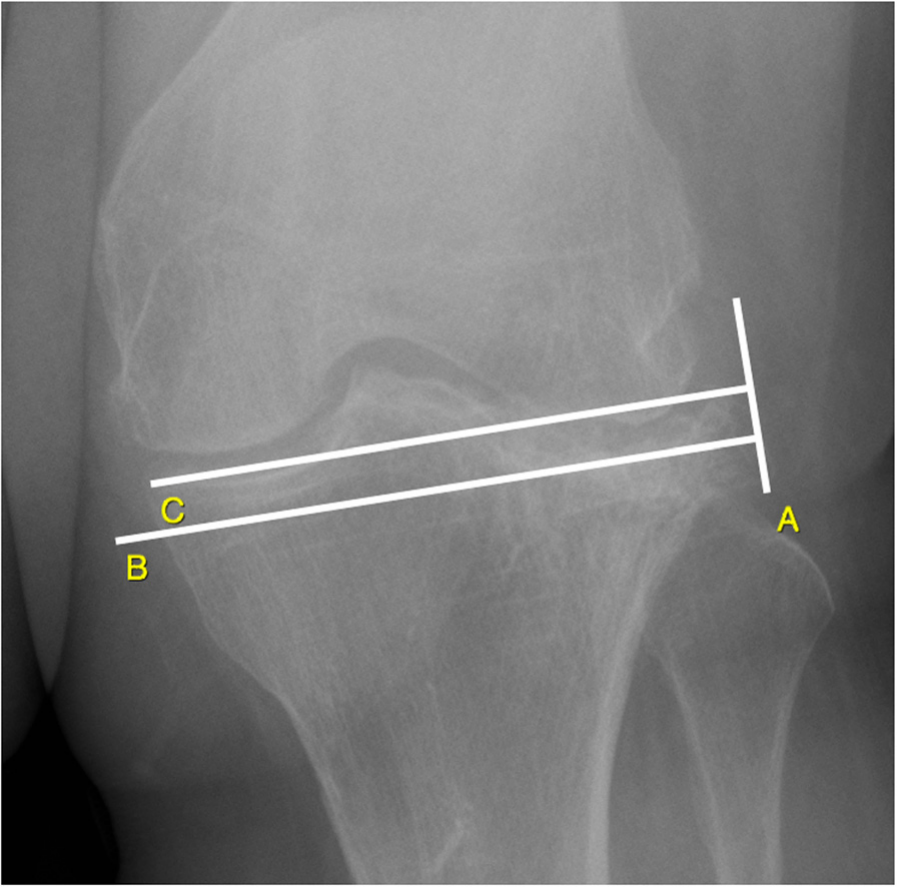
Method of measurements on plain radiograph anteroposterior (left knee – affected side): Lateral plateau impression is the difference between line (b) drawn from the maximum impression of tibial plateau baseline parallel to line (c) that is drawn in extension of the medial plateau parallel to the joint line. Line (a) is the common perpendicular to lines (b) and c. There was valgus alignment of the knee joint (not shown)
